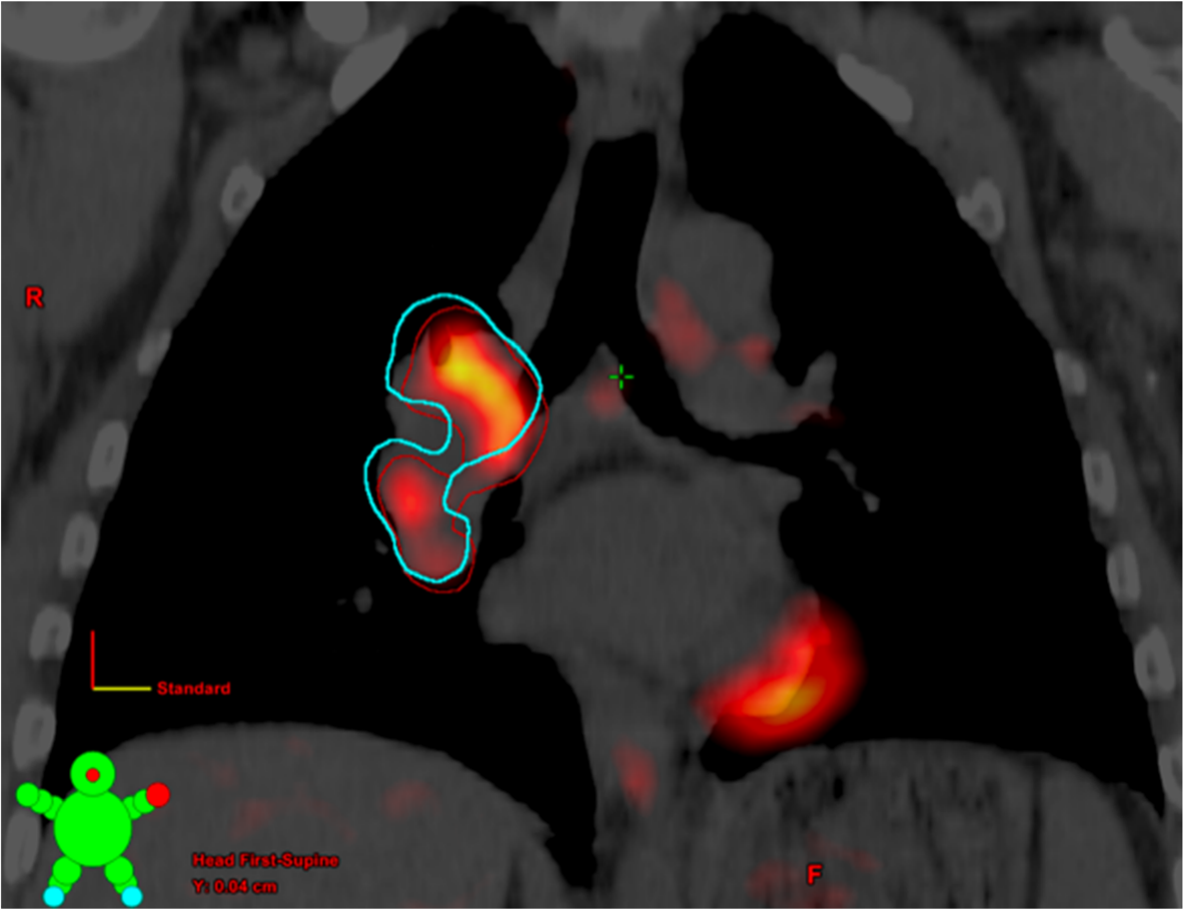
Coronal view of the planning CT with fused PET for a lung cancer subject. The GTV contours generated using the RIR and DIR PET images are shown in red and blue, respectively. An intensity threshold equivalent to SUV ≥ 2.0 was used for the PET image.
